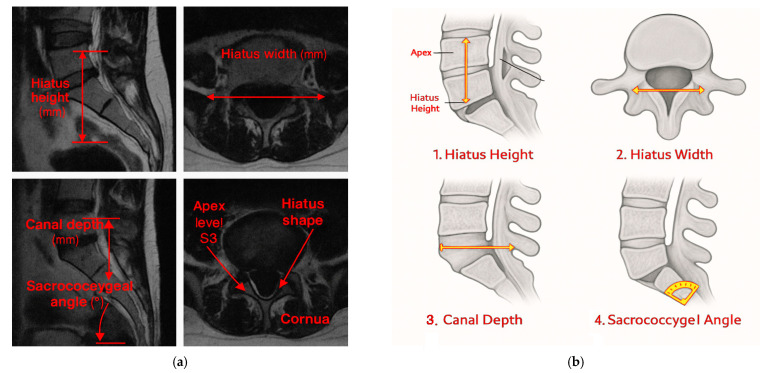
Anatomical measurement parameters pertaining to the sacral hiatus (MRI Sections). (a) Sagittal and axial MRI views demonstrating key morphometric parameters: hiatus height, hiatus width, canal depth, sacrococcygeal angle, apex level (S3), hiatus shape, and the presence of cornua. (b) Definitions: hiatus height and width, canal depth, sacrococcygeal angle, apex level, morphology, the presence of cornua. Abbreviations: MRI, Magnetic Resonance Imaging.
